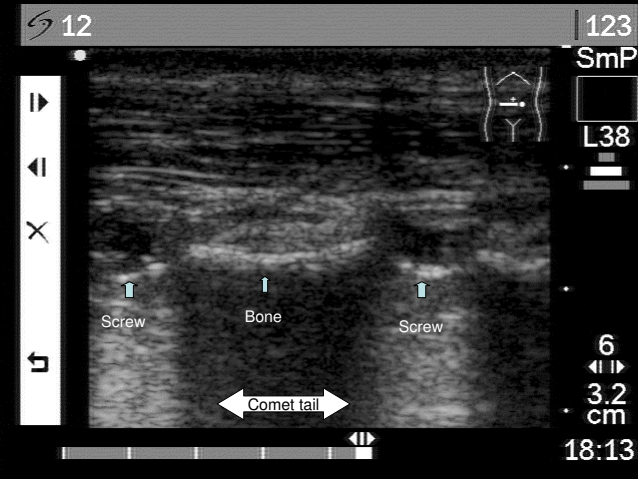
Sonography of screws. Two distal locking screws can be visualized by sonography. The image of two extremely brightness with "comet-tails" indicate the distal locking screw heads.
